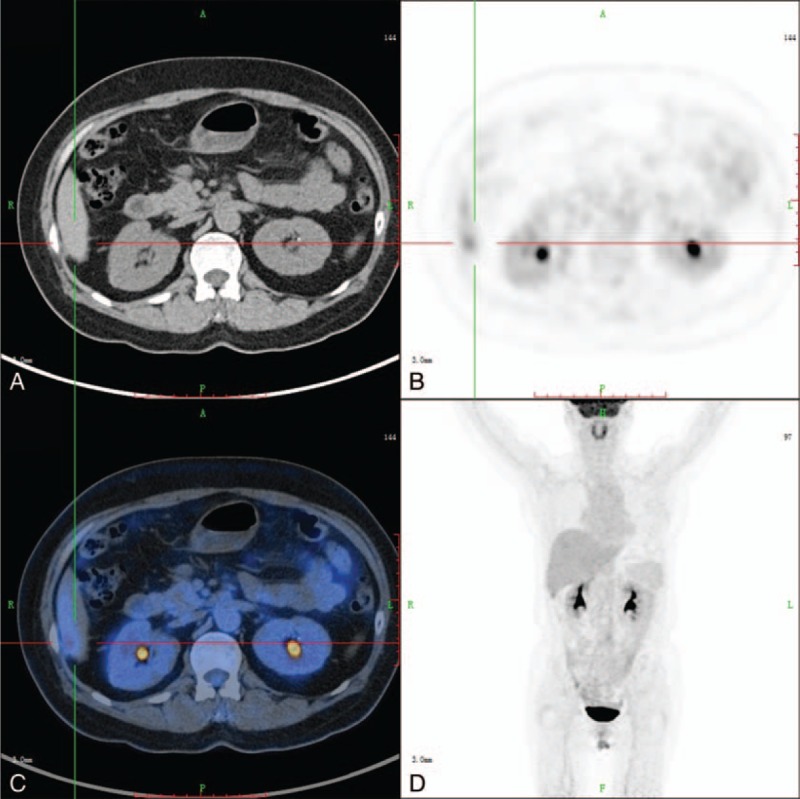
Images of 70-year-old woman who presented with abdominal pain for 6 months: axial CT (A), axial PET (B), axial fused PET/CT (C), and 3D PET (D). CT examinations could not detect the hepatic peritoneal metastasis from ovarian cancer. PET/CT images show high uptake in the hepatic peritoneum (SUV_max_ of 3.5). Metastasis in the hepatic peritoneum was finally confirmed by pathology. CT = computed tomography, PET = positron emission tomography, SUV_max_ = maximal standardized uptake value.
